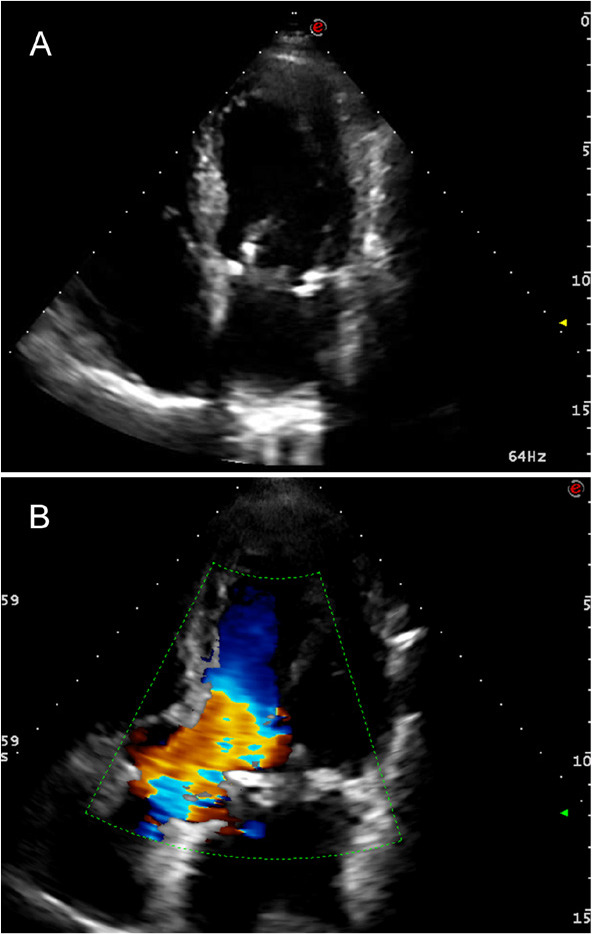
Postoperative transthoracic echocardiographic images demonstrating
